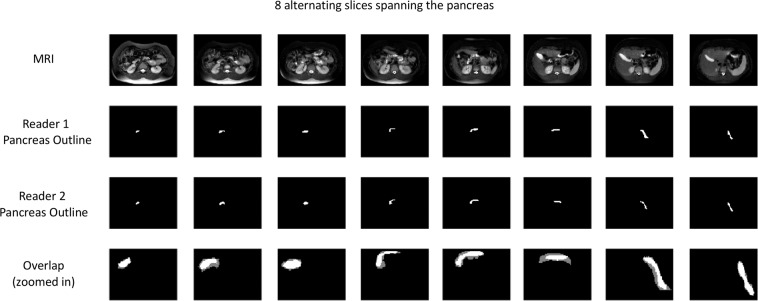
Outlines of pancreas by two readers of the same images overlap. The pancreas from a representative subject (Dice coefficient = 0.77) was outlined by two board-certified radiologists across eight alternating MRI slices of the pancreas. The overlap between the two readers’ pancreas outlines is shown in the bottom row and zoomed in for clarity. Regions of agreement are shown in bright white, while gray regions denote regions of non-overlap. The average Dice coefficient was 0.77 for the 31 scans analyzed.
